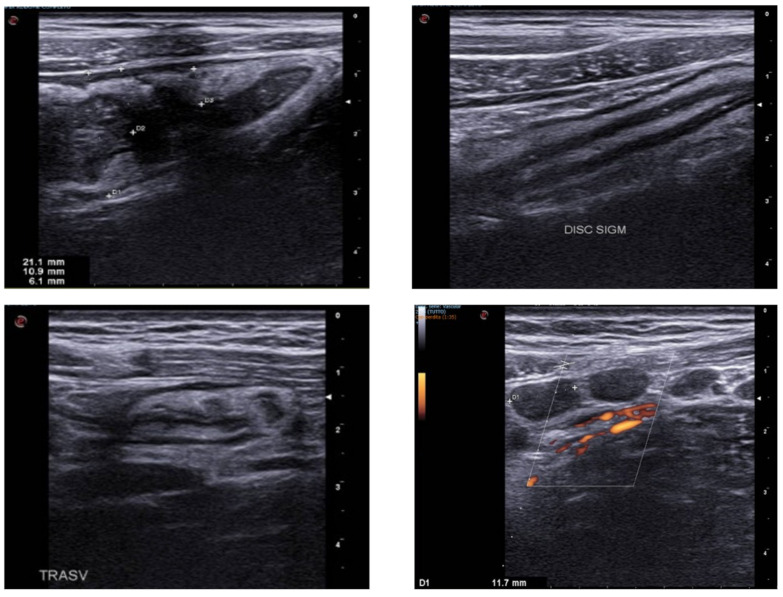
Patient went to the emergency room reporting diffuse abdominal pain for about 10 days associated with diarrhea and traces of blood and mucus, and weight loss (about 10 kg). GIUS showed diffuse wall thickening of the entire colon, particularly at the level of the ileocecal valve (11 mm) and submucosa thickness of 6 mm; multiple lymph nodes were evident in the right iliac area, with a maximum size of 11 mm. The clinical and ultrasound findings are compatible with Crohn’s disease.
